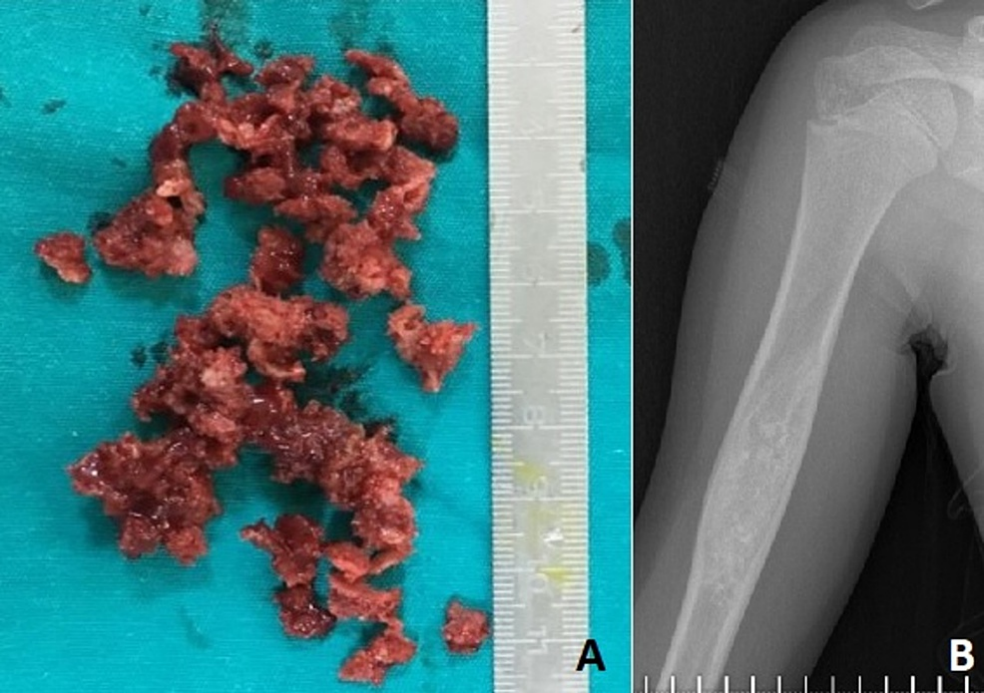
Macroscopic view of the excised lesion (A); Postoperative third-month control X-ray after curettage and bone grafting (B).
